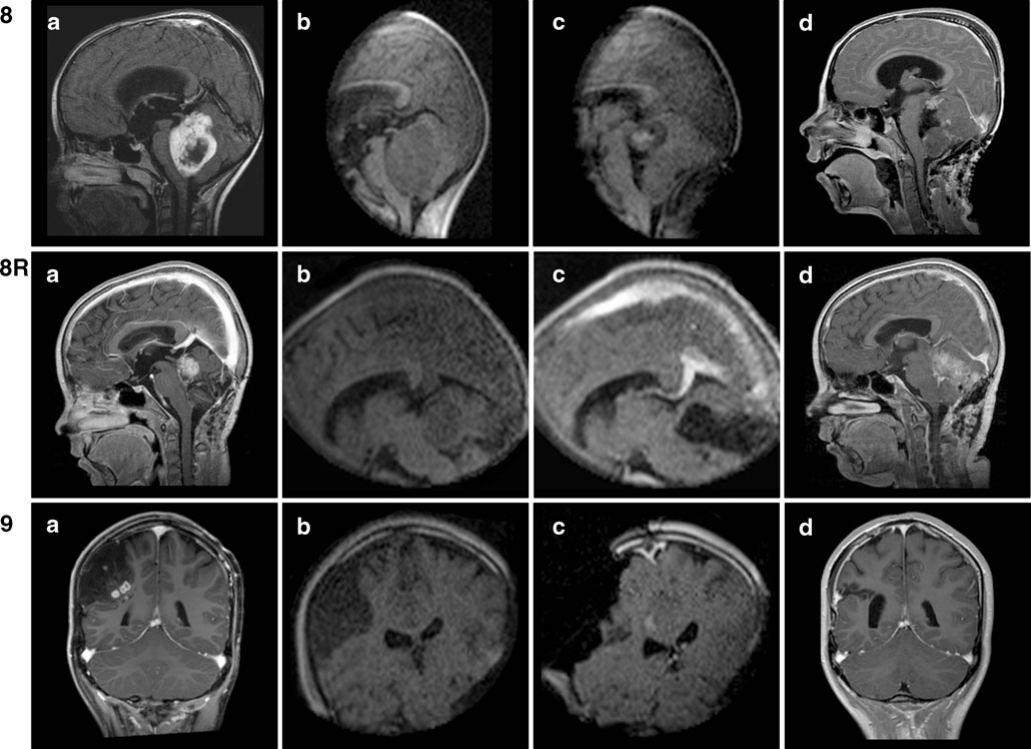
Overview of preoperative (a), intraoperative (b), postresection intraoperative (c), and early postoperative MR images (d) in all cases (*n* = 11). The intraoperative images are T1 7-min 4-mm sequences (preresection without contrast, postresection with contrast). The postoperative images are T1 sequences with contrast
